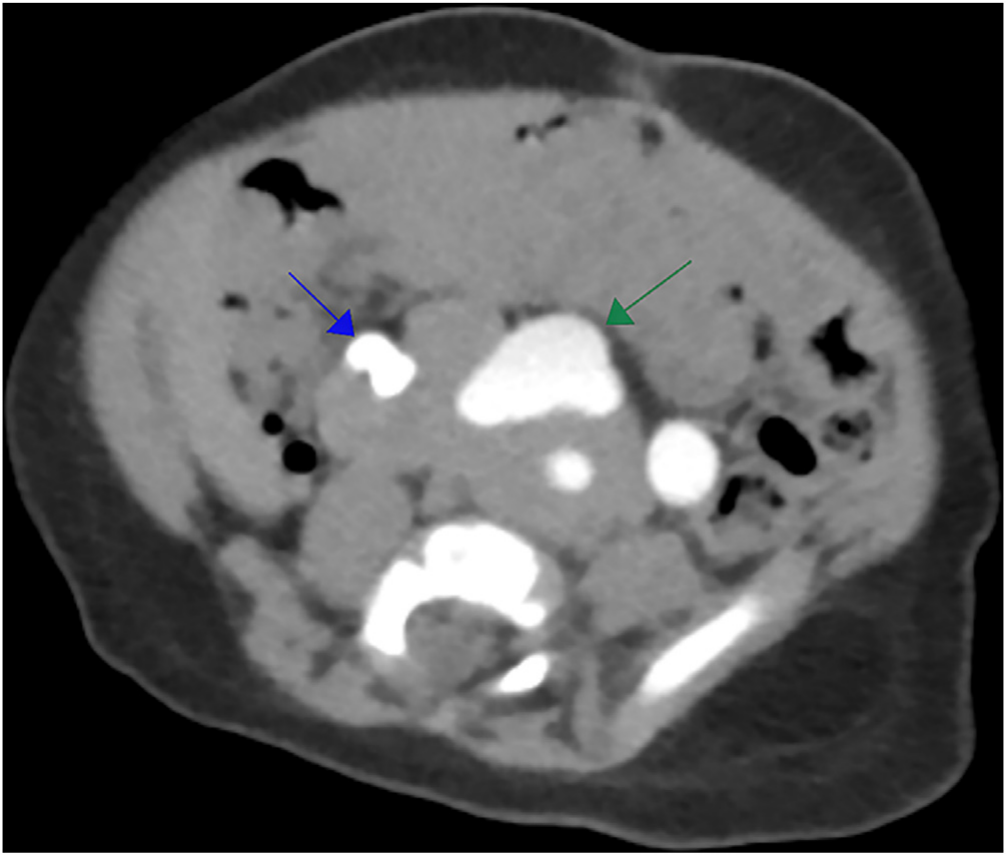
CT urography reveals the abnormal positioning of both kidneys. The right pelvicalyceal system is malrotated with no signs of dilation (blue arrow), whereas the left pelvicalyceal system is malrotated with moderate dilation, suggesting a PUJ obstruction (green arrow).
